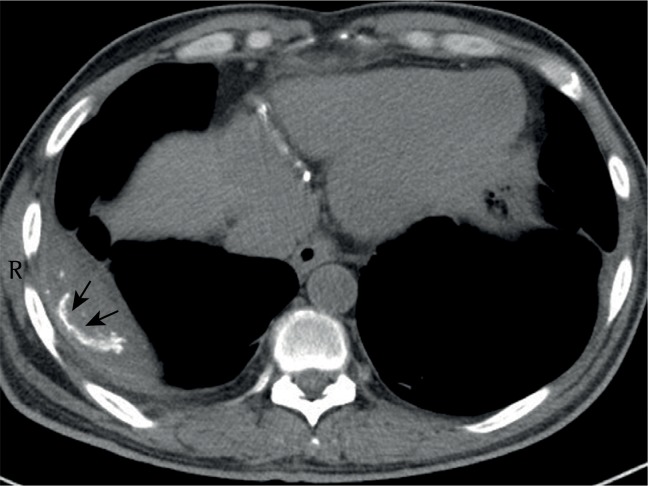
Non-contrast-enhanced chest CT scan with mediastinal window settings.
